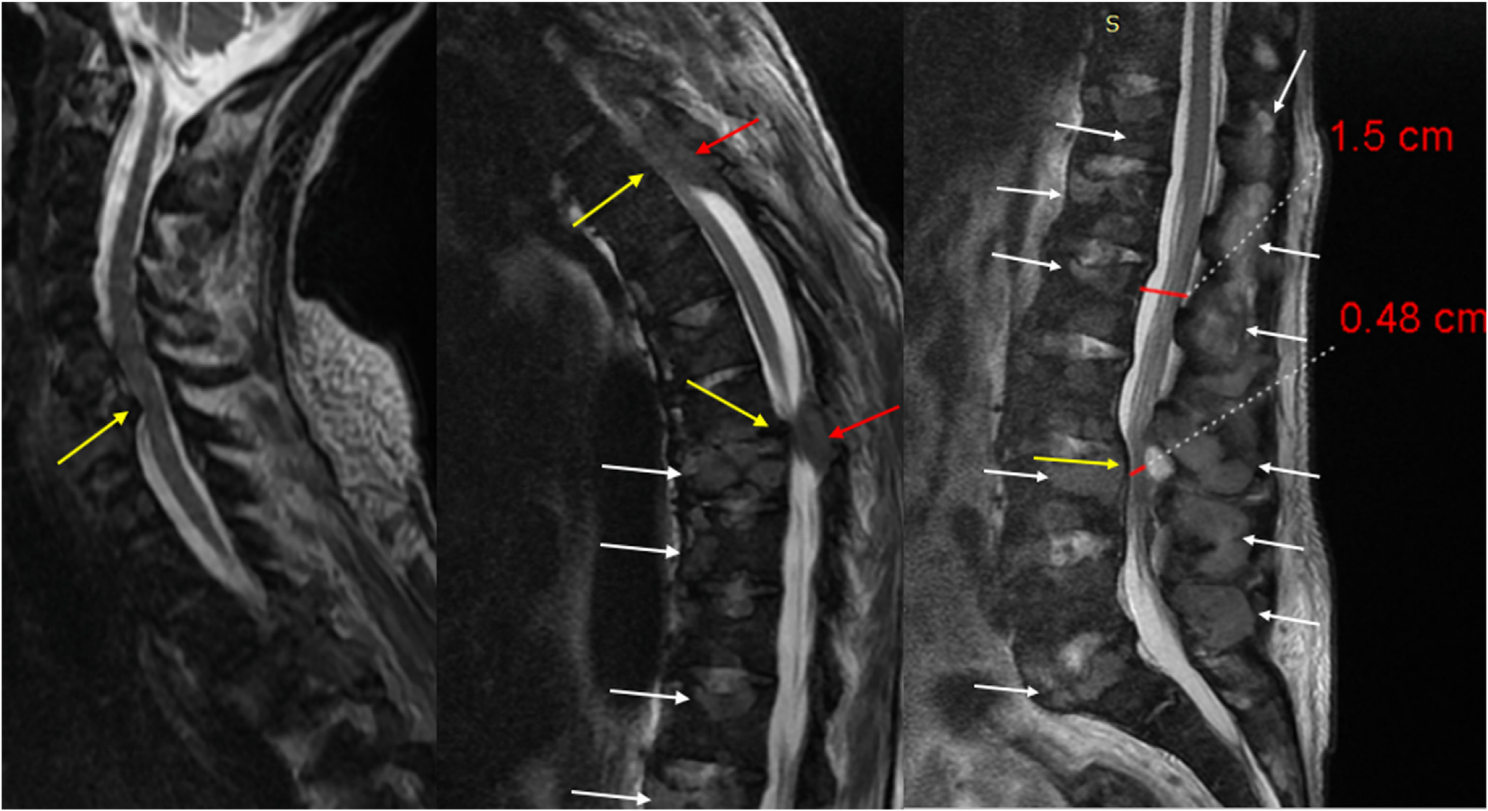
MRI (cervical, thoracic, and lumbar) without contrast; T2 sequence shows the following:1)Vertebral and spinous processes deformities and compressions (white arrows).2)Spinal cord compression and spinal canal stenosis on cervical, thoracic, and lumber spine (yellow arrows)3)Epidural mass effect on T4, T9 (red arrows).
